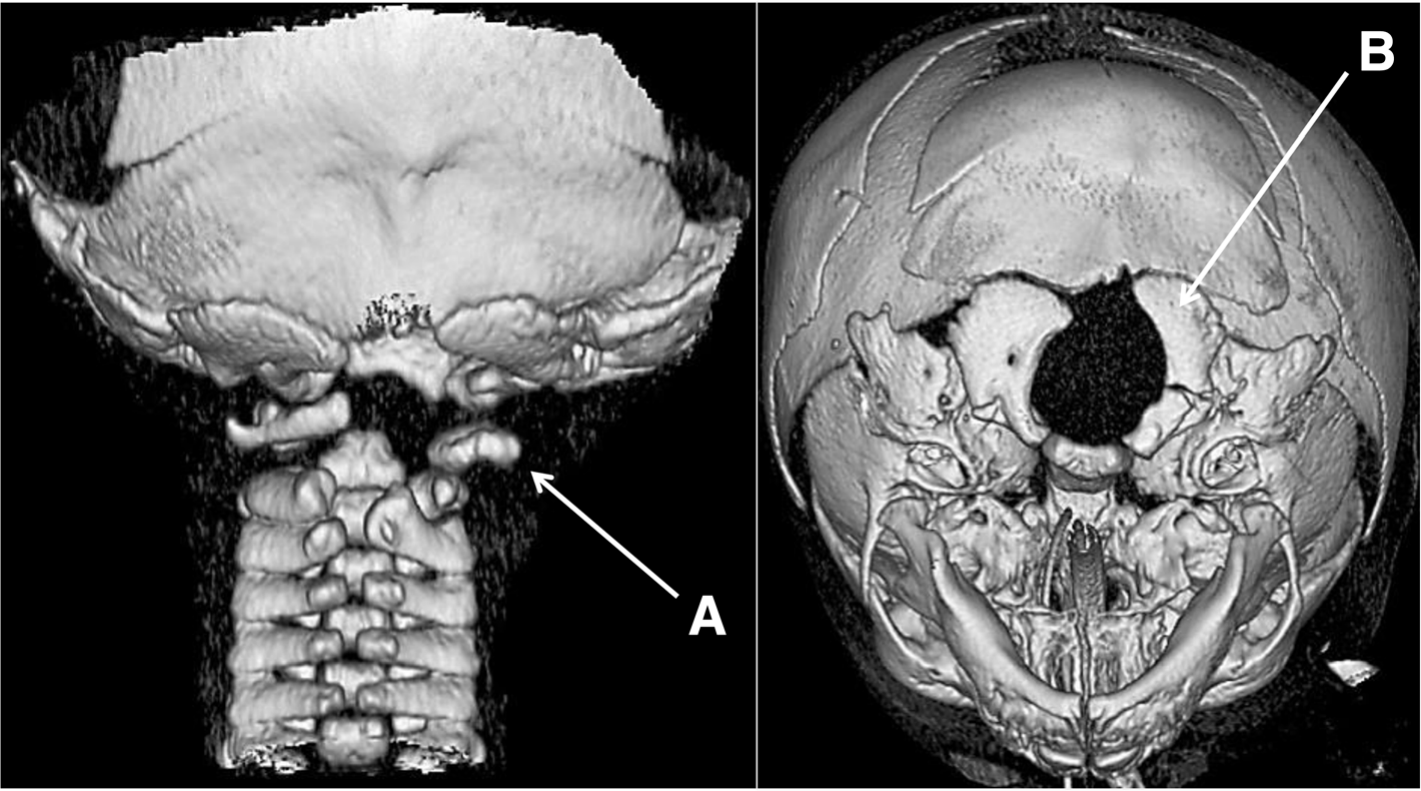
3D bone CT reconstruction showing hypoplasia of the posterior hemiarches of C1-C2 (a) and the right exo-occipital bone (b) and a small clivus
